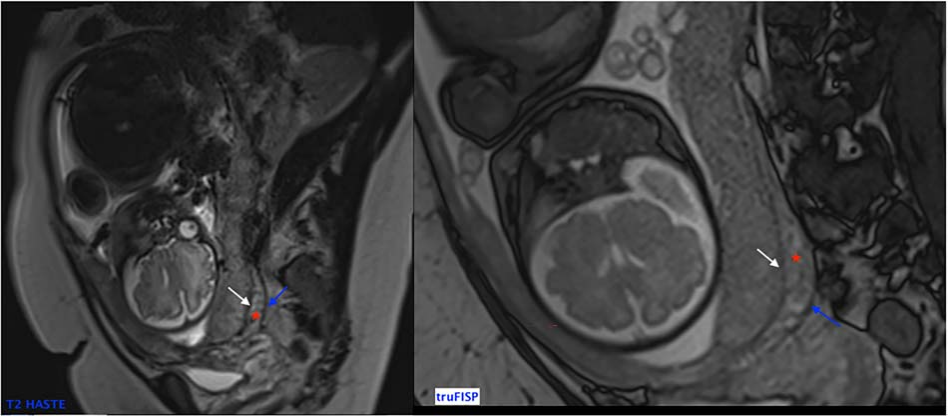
Sagittal T2 Weighted Images showing normal MRI signal of the placenta and the myometrium at the end of 2nd trimester. Note the trilaminar aspect of the myometrium: retro placental layer in hyposignal (white arrow), intermediate layer (the zone of arcuate vessels in intermediate signal red asterisk) and the external layer (the serosa) with a thin T2 hyposignal aspect (blue arrow).
